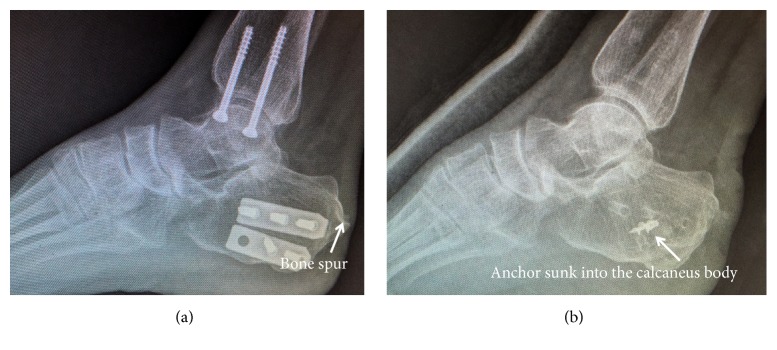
Case 4, a 61-year-old woman showed right posterior heel pain for 1 year, and she also underwent calcaneal and medial malleolus medialis fracture 1 year before the symptom*. Due to osteoporosis and inappropriate force during operation, the anchor sank into the calcaneal body, causing the decreased pullout strength of anchor. (a) Preoperative radiograph showed the bone spur near the posterior calcaneus and osteoporosis sign. (b) Postoperative radiograph demonstrated the anchor had sunk into the calcaneus body.
